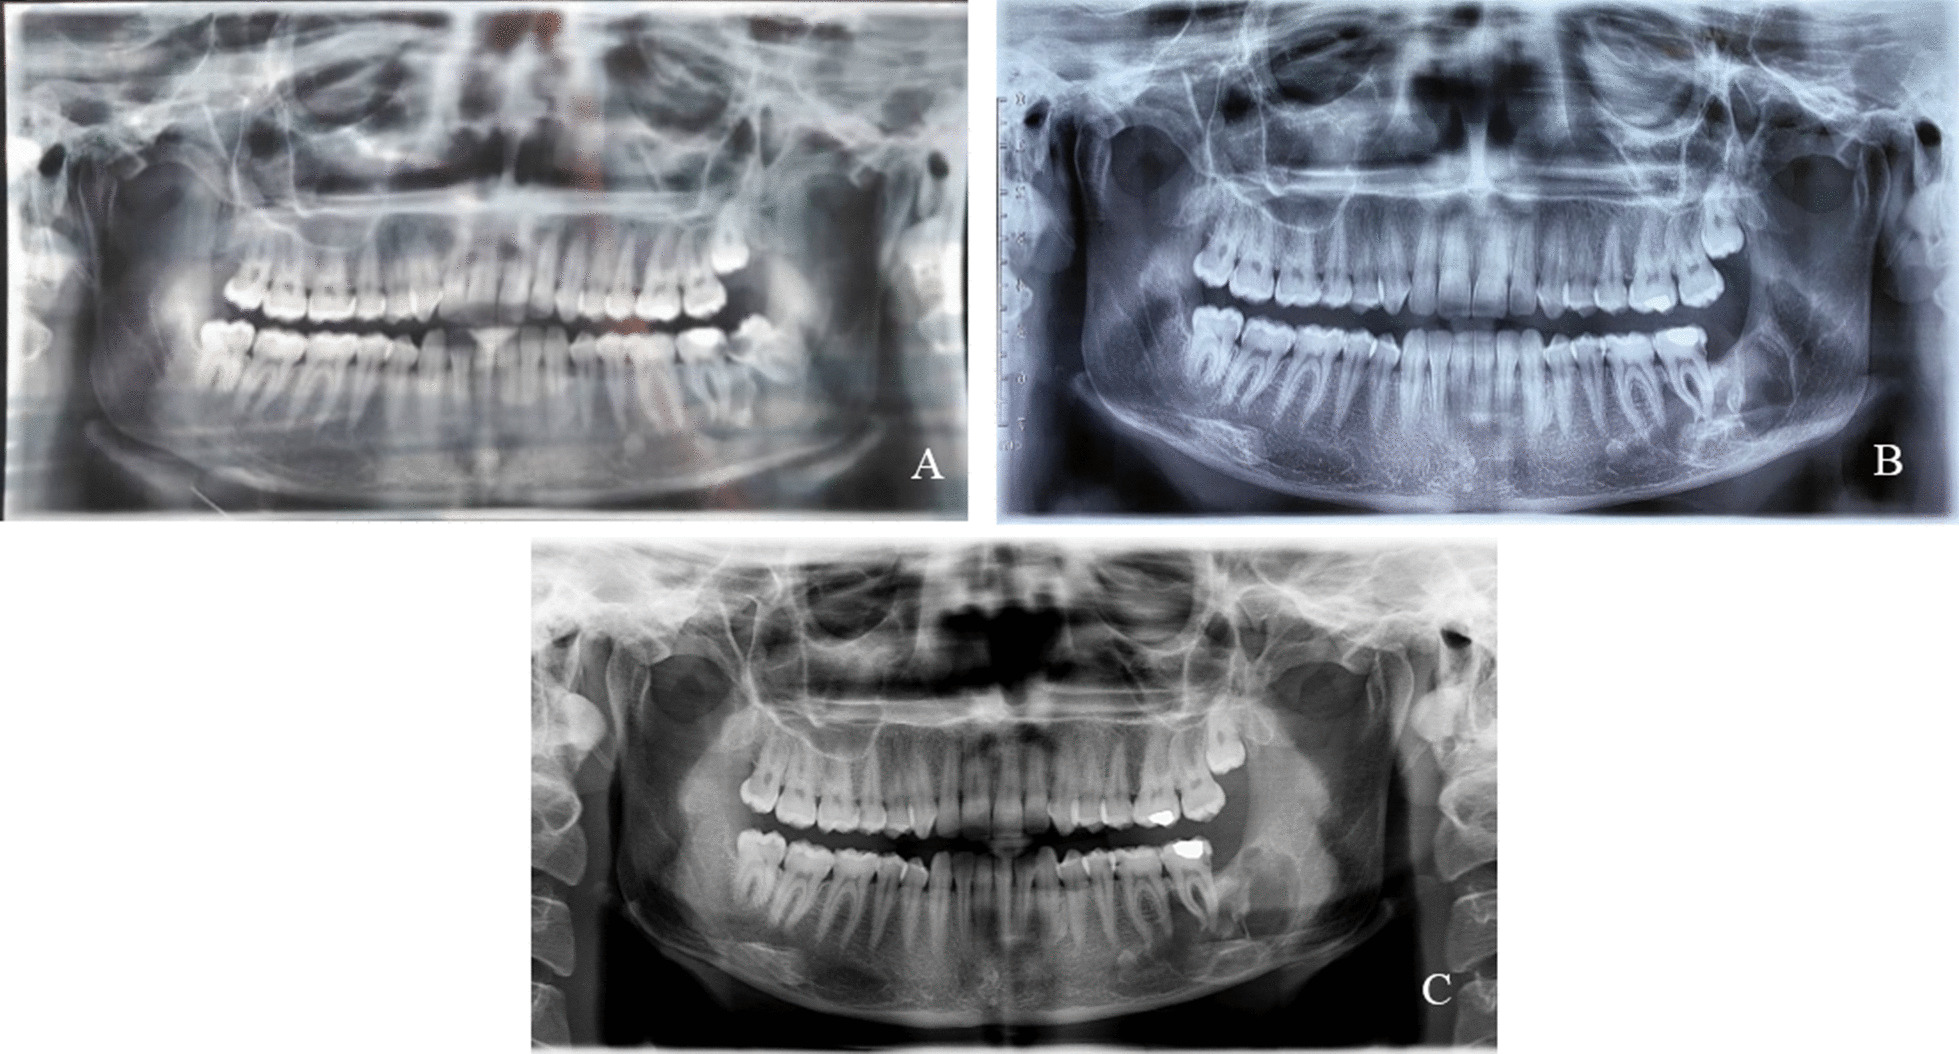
A Orthopantomography demonstrating multilocular radiolucency, B Orthopantomogram taken 3 months post-operatively. The lesion site is undergoing a healing process, C Orthopantomogram taken 6 months post-operatively. Notice the healing process and shrinkage of the lesion
